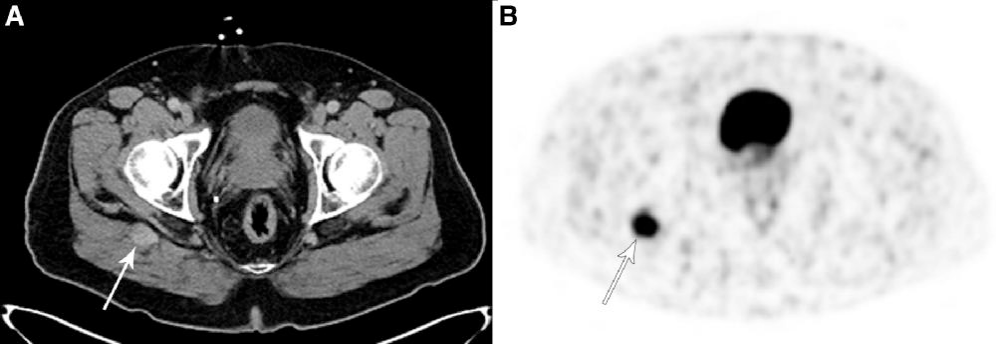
(A) Non-contrast CT scan taken at time of PET scan for attenuation correction to identify anatomic location of ^68^Ga-DOTATATE radiopharmaceutical. A 2.2-cm mass is present deep within the right gluteus maximus muscle (arrow); (B) ^68^Ga-DOTATATE PET scan showing intense (SUV = 11.2) uptake in the mass (arrow).
